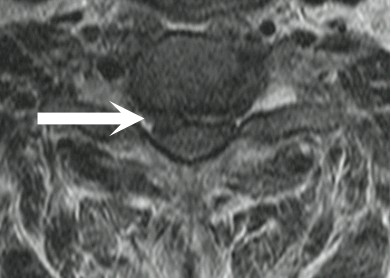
Axial T2 weighted MRI reveals a bi-lobed disc herniation (arrow), with secondary spinal canal encroachment and mass effect on the cervical spinal cord at the C6-7 level.
